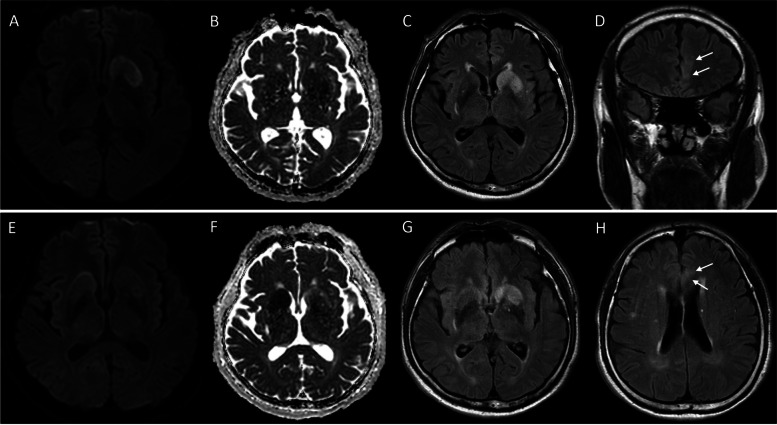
Brain MRI findings at 1st (A-D) and 2nd admission (E-H). On the first admission, the left caudate nucleus and putamen showed high signal intensity on DWI (A) and low signal intensity on the ADC map (B). Hyperintense lesions on FLAIR were detected in the left caudate nucleus and putamen (C). Acute lesions on the contralateral side were detected on the second admission (E, F, G). A slightly high signal intensity (arrow) on FLAIR was detected in the left medial frontal lobe (D, H)
